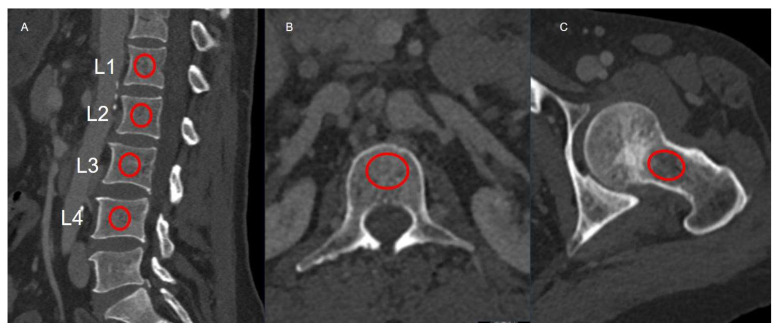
Sagittal lumbar (A), axial lumbar (B), and femoral neck (C) CT images demonstrating regions of interest (red circles) used for HU measurements. The ROIs shown are for demonstration purposes only; actual quantitative measurements were performed using workstation-defined ovoid ROIs with a diameter of approximately 2–2.5 cm.
